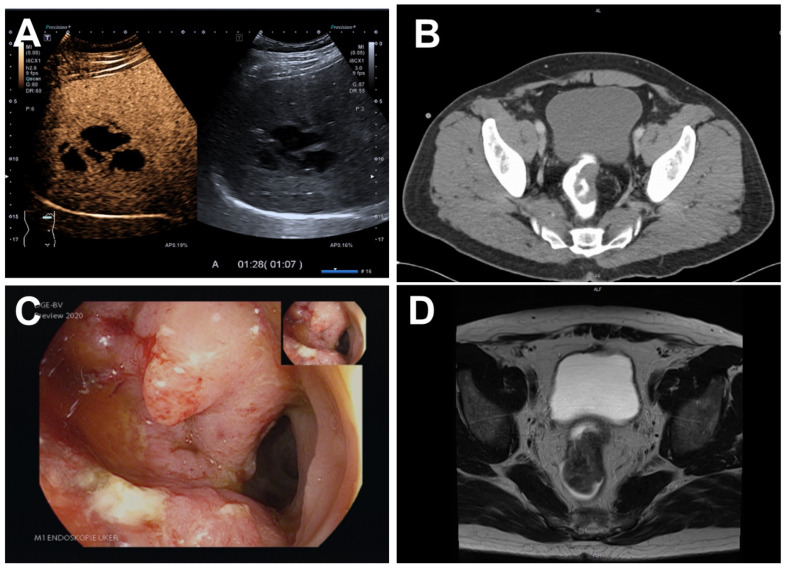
(A) Contrast-enhanced ultrasound of the liver abscess in the portal venous phase. (B) Initial CT scan revealing a tumor in the rectum. (C) Endoscopy of the tumor in the rectum. (D) Initial MRI scan of the tumor in the rectum.
